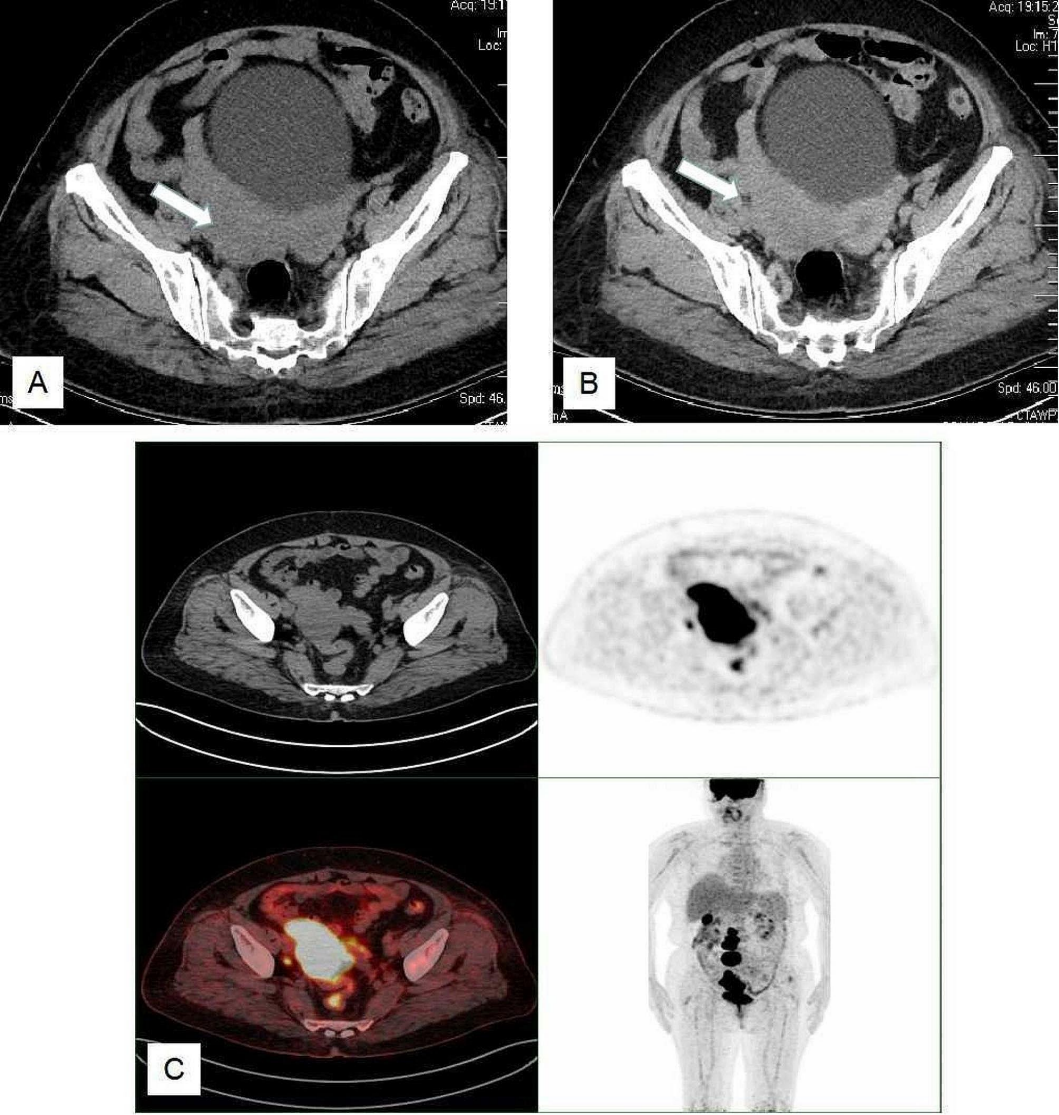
A. CT images: The lesion of the right ovary is demonstrated on the axial scan of the CT, and the size is about 45 mm*81 mm. B. Axial contrast-enhanced CT images show medium enhancement. C. PET-CT images: The PET images of axial view show the uptake in the right ovary lesion and enlarged lymph nodes in the pelvic cavity. Fused images of PET-FDG show the lesion of the right ovary and enlarged lymph nodes with increased uptake. The PET images of coronal view show the uptake in the right ovary lesion and enlarged lymph nodes of the retroperitoneum (Case 11)
